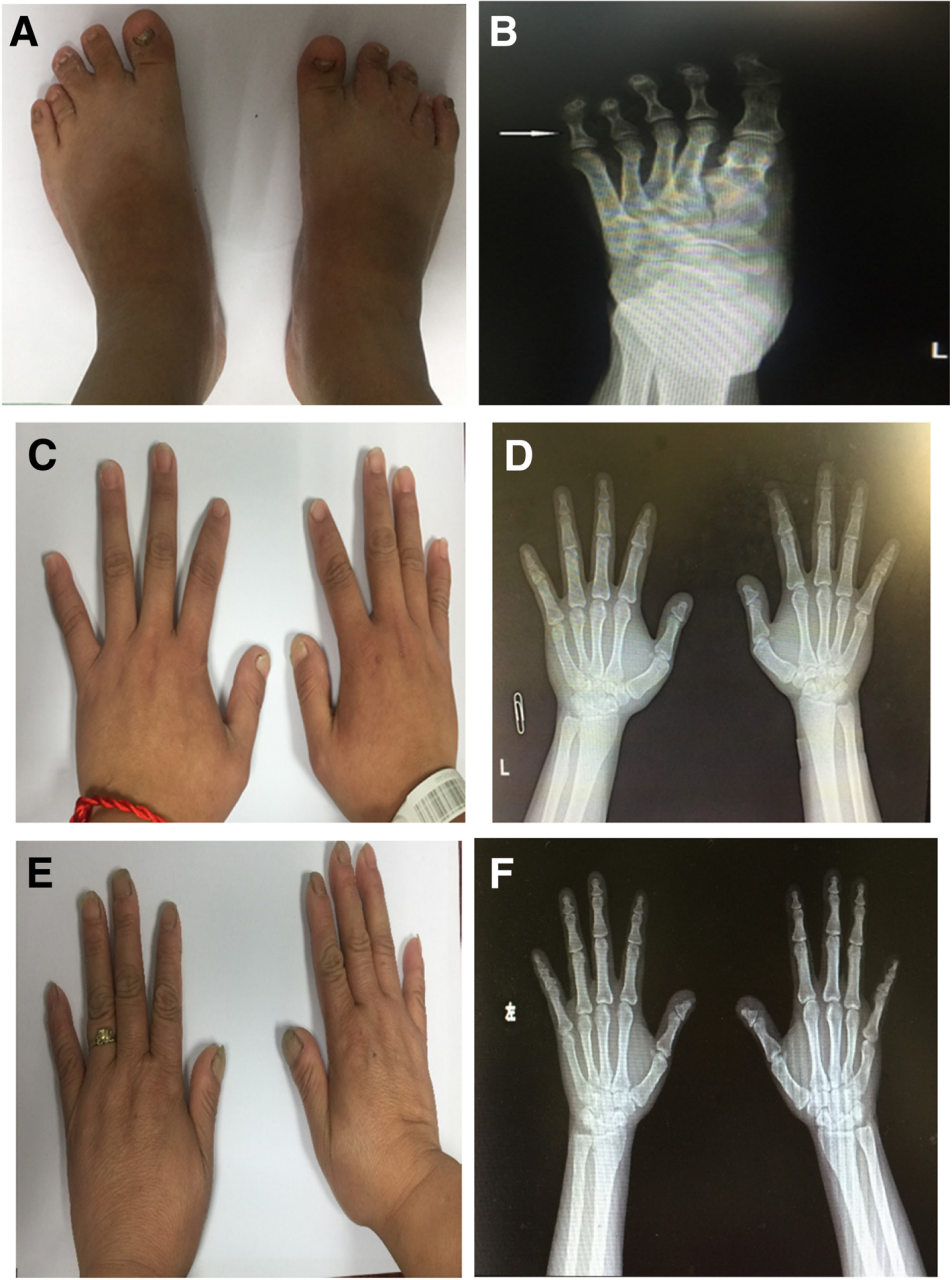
(a) Photograph of the patients feet and (b) X-ray of the left feet showing brachymetatarsia. (c) Photograph of the patient’s hands and (d) X-ray of her hands (d) showing brachydactyly, typical of Albright’s osteodystrophy. (e) Photograph of the patient’s mothers hand and (f) X-ray of her hands (f) also showing slight brachydactyly similar to her daughter
